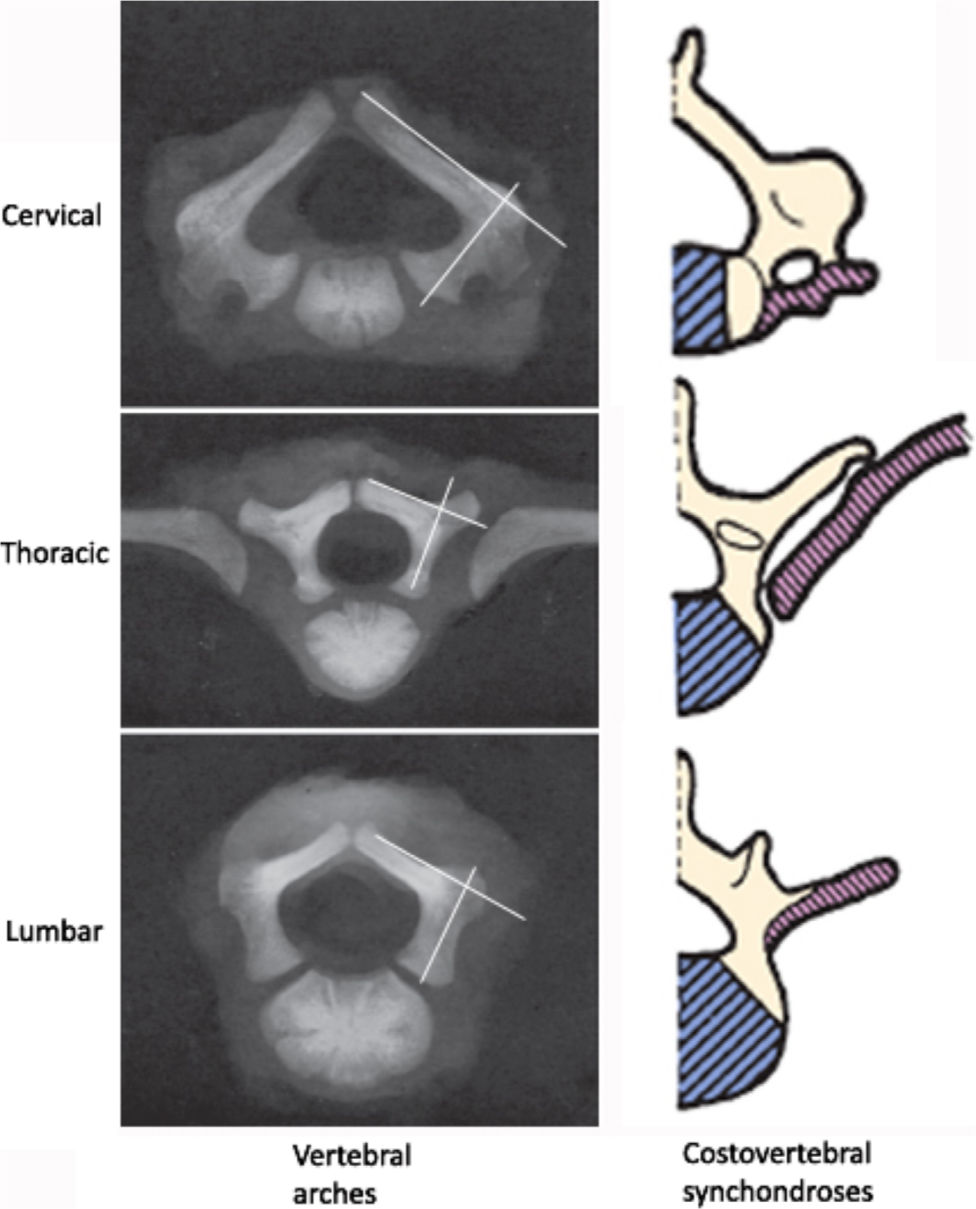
Development of the pedicles. The laminar of the isthmus and pedicle are developed from ossification of the same vertebral arches on a superior radiograph (left column). Costovertebral synchondroses developed a true rib and diarthrodial joint in the thoracic region, and the transverse process was observed in the lower cervical and lumbar vertebrae (right column).
